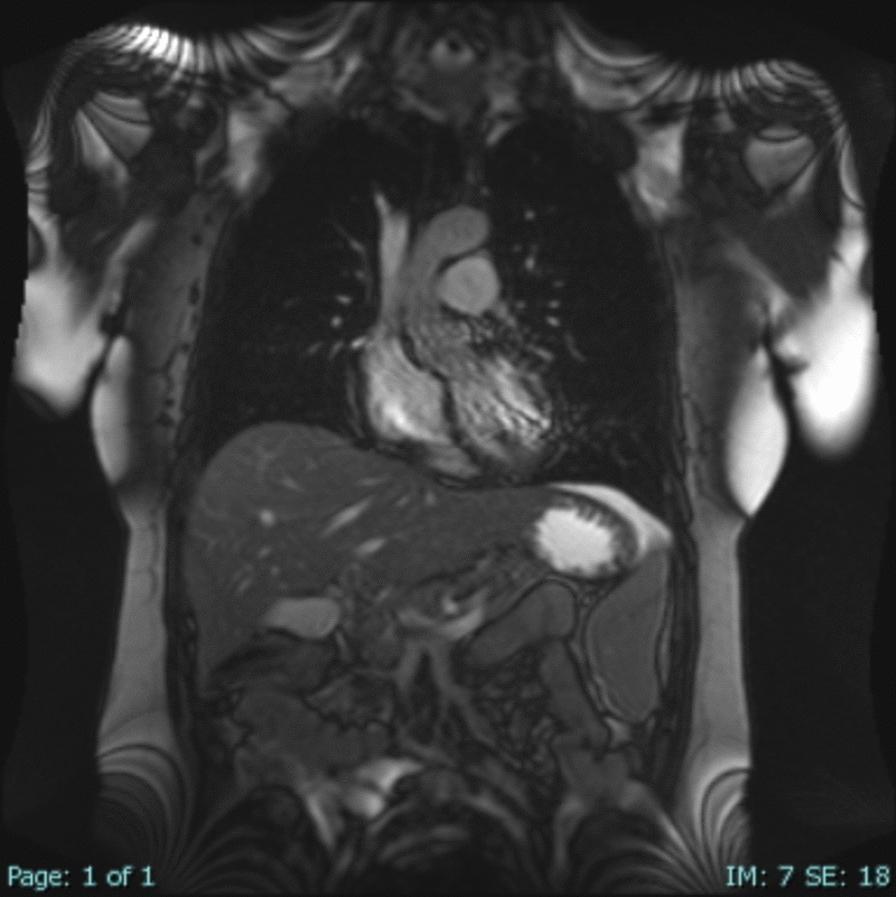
Postoperative dynamic sagittal chest magnetic resonance imaging demonstrating a symmetrical diaphragm on both sides, without evidence of elevation or re-rupture on the right side
